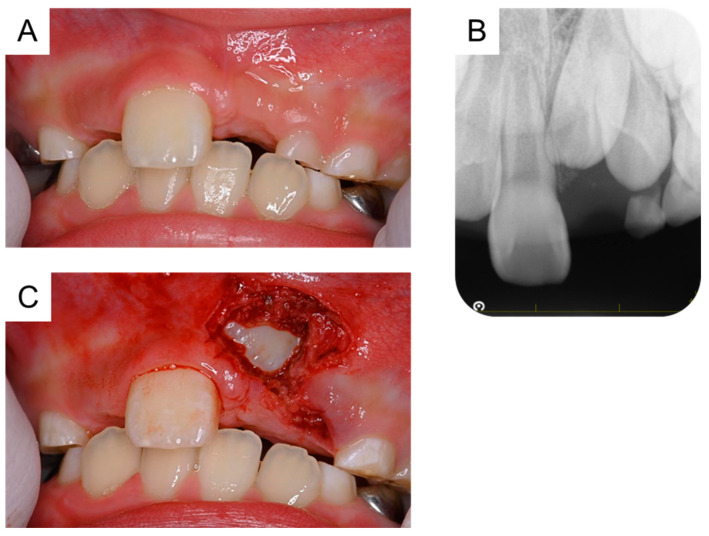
Fenestration of the maxillary left central incisor. (A) Intraoral photograph and (B) periapical radiograph after the extraction of the third supernumerary tooth at the age of 8 years and 9 months. (C) Intraoral image just after fenestration of the maxillary left central incisor at the age of 8 years and 9 months.
